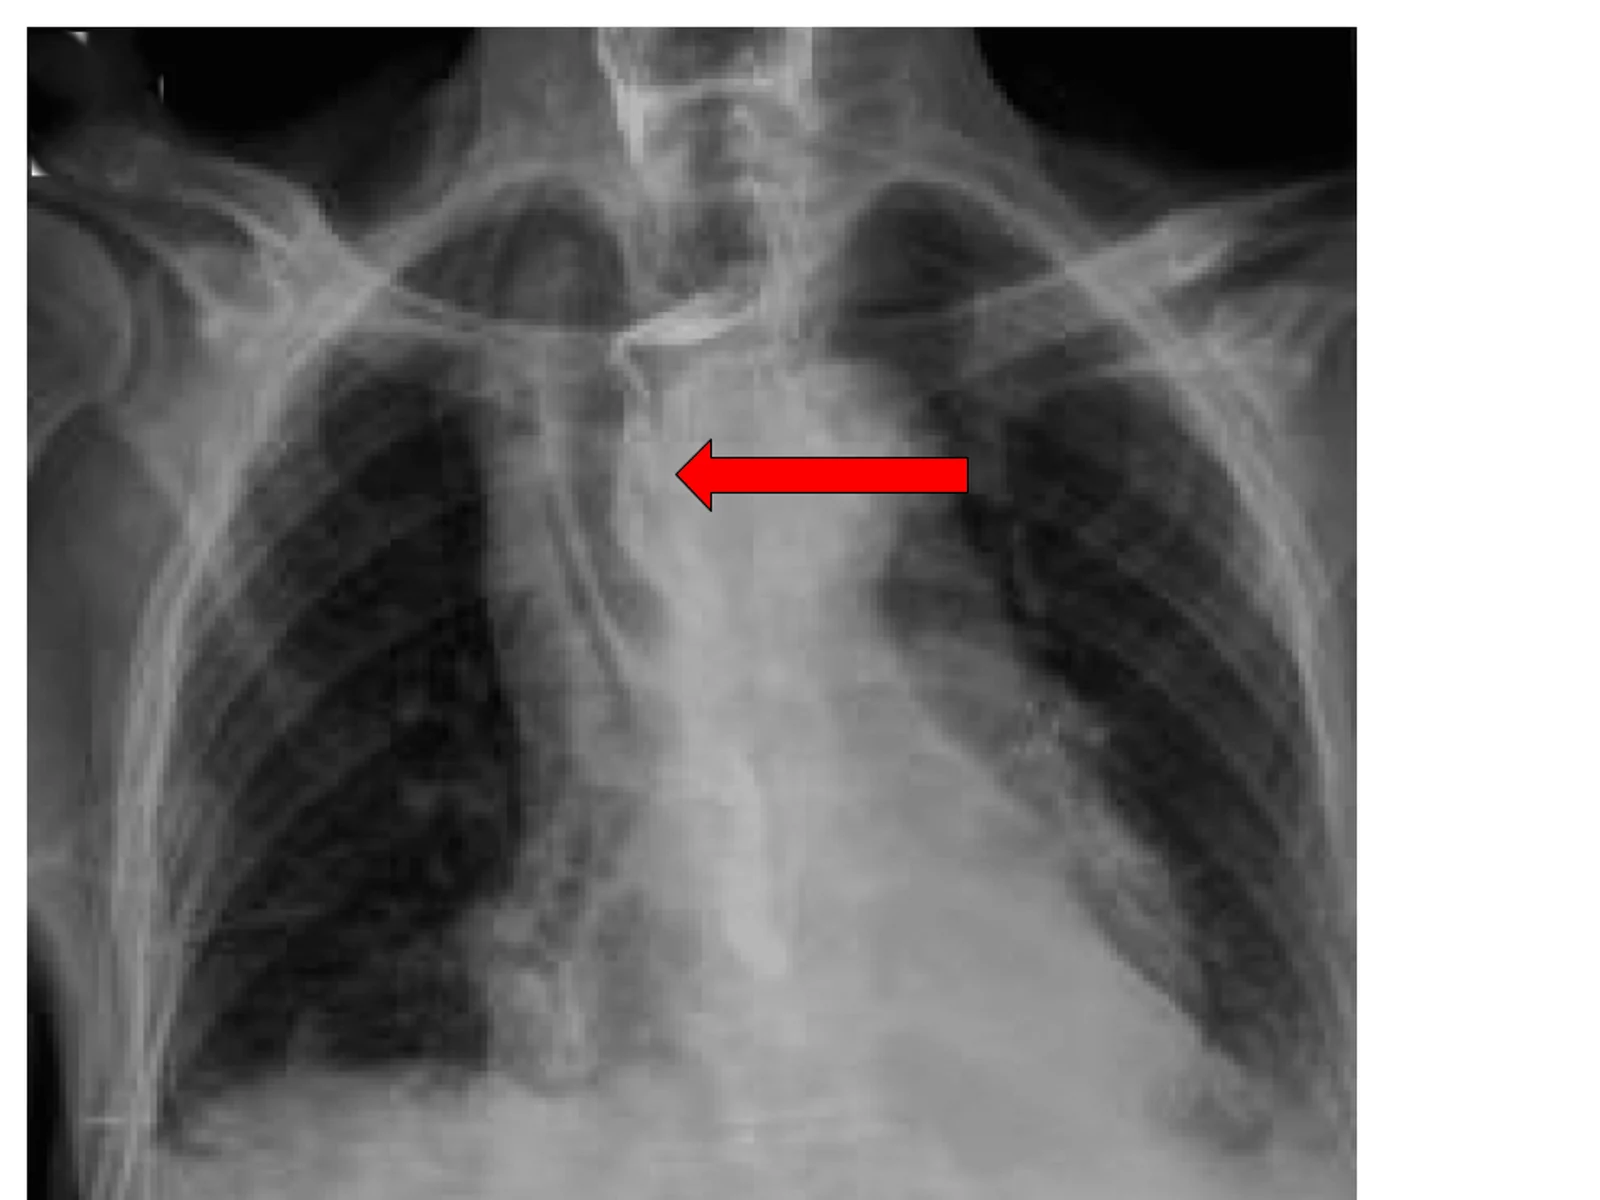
Frontal chest radiograph of the esophagus The esophagus is displaced to the right by the mediastinal hematoma
